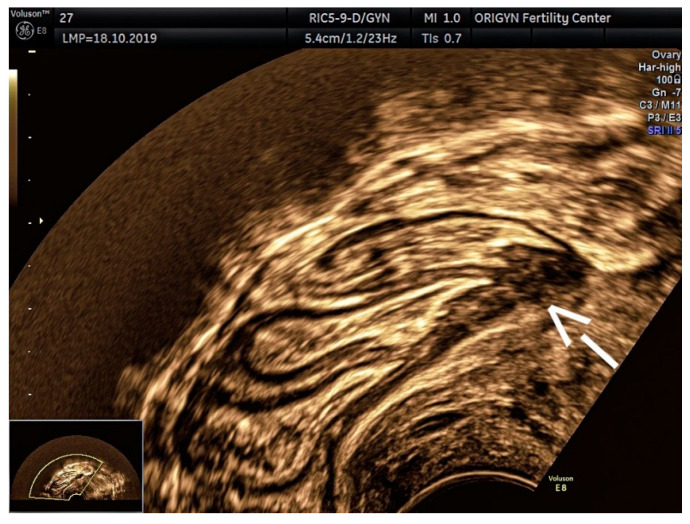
Ultrasound image showing the bowel with an endometriotic nodule (white arrow, “pulling sleeve sign”) adherent to the uterine torus, in extrinsic retraction. In this case the sliding sign was absent.
